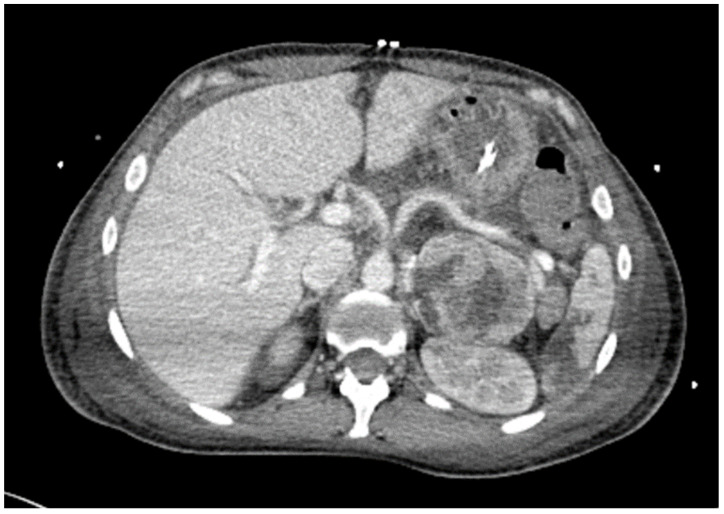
The Emergency CT scan showed a giant left adrenal tumor.
